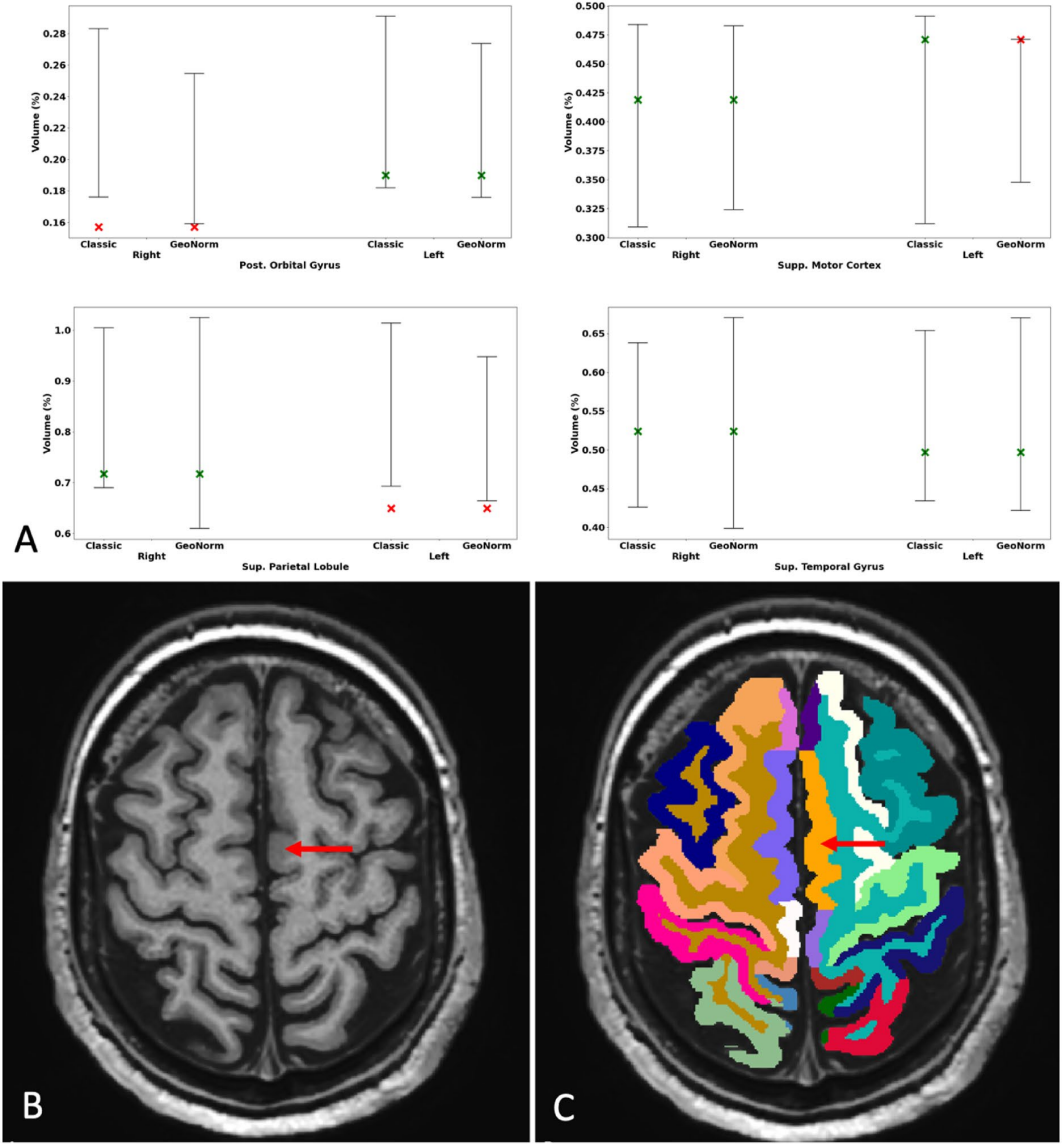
Normative range for the % volume of four structures for the 47-year-old randomly selected patient with FCD in the left supplementary motor cortex (A, Subject 25). The variation between normative ranges depends on the anatomical area. For example, the LifeSpan-based normative values placed the patient’s left supplementary motor cortex in the upper end of the range but still within the normal range (green cross), while GeoNorm considered this structure outside of the personalized normative values (red cross). Both models also found the left superior parietal lobule as atrophic, but this was less severe using GeoNorm. The Presurgical T1-weighted imaging (B) and AssemblyNet segmentation (C) are shown in the bottom row. For example, for the left supplementary motor cortex, AssemblyNet classified it as normal, while the GeoNorm personalized normative values classified it as with increased volume.
